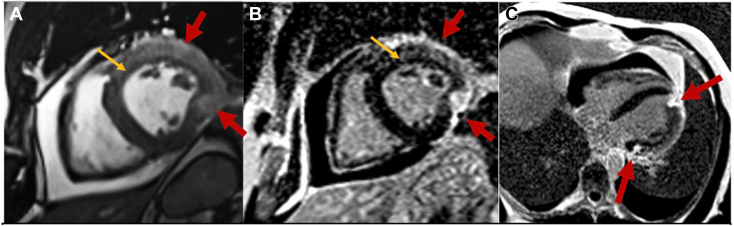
(A) CMR, T2-weighted short-tau inversion recovery short-axis image, demonstrates increased signal throughout the anterior and lateral walls (*red arrows*), sparing the subendocardium (*yellow arrow*), consistent with myocardial edema. (B) CMR, LGE sequence using phase-sensitive inversion recovery (PSIR), short-axis image, demonstrates subepicardial enhancement in the same distribution (*red arrows*) with subendocardial sparing (*yellow arrow*). (C) CMR, LGE sequence using PSIR, axial view, demonstrates thickening of the lateral wall of the left ventricle with more intense delayed enhancement along the lateral wall at the base and inferolateral mid and apical wall where the myocardium appears pinched, suggesting ring-shaped constriction with associated LGE.
